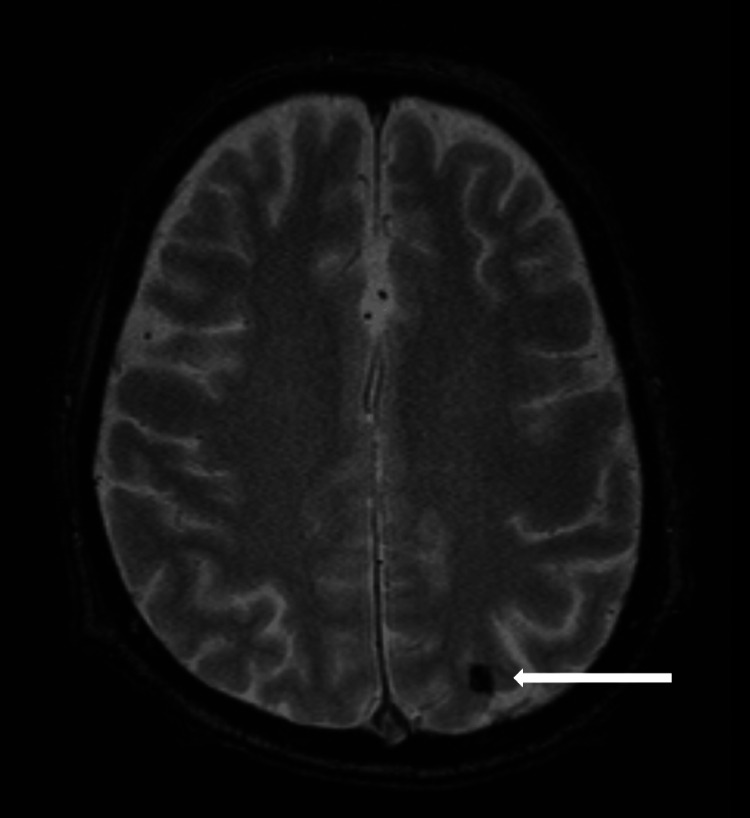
T2*-weighting effect on MRI A hypointense spot is observed in the left parietal lobe (white arrow). MRI, Magnetic resonance imaging
